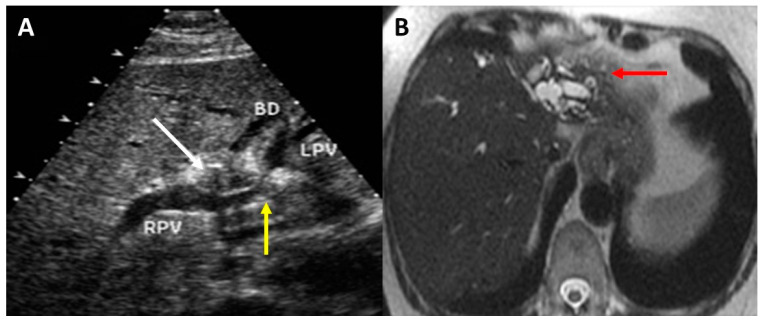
Lobar atrophy associated with perihilar cholangiocarcinoma. Preoperative ultrasonography (A) demonstrating perihilar biliary mass (white arrow) with resultant narrowing of the left portal vein (yellow arrow). MRI (B) demonstrated substantial left lobar atrophy (red arrow).
